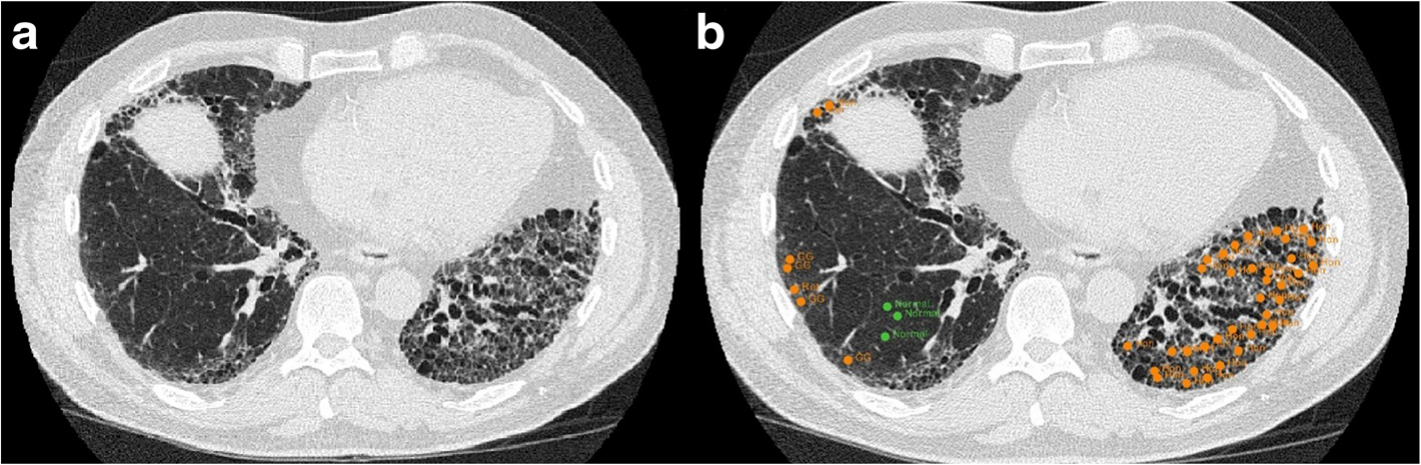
a Sample slice for CT scan of a subject. b The same sample slice from a subject CT scan showing placement of fiducials for the training of the local histogram based objective method. Abbreviations: ground glass (GG), honeycombing (Hon), reticular (Ret), computed tomography (CT)
